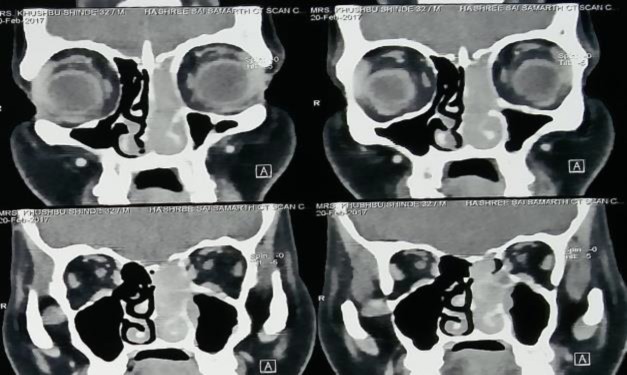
Contrast enhanced CT scan, coronal view showing contrast enhanced soft tissue mass in left nasal cavity with cribriform plate erosion
